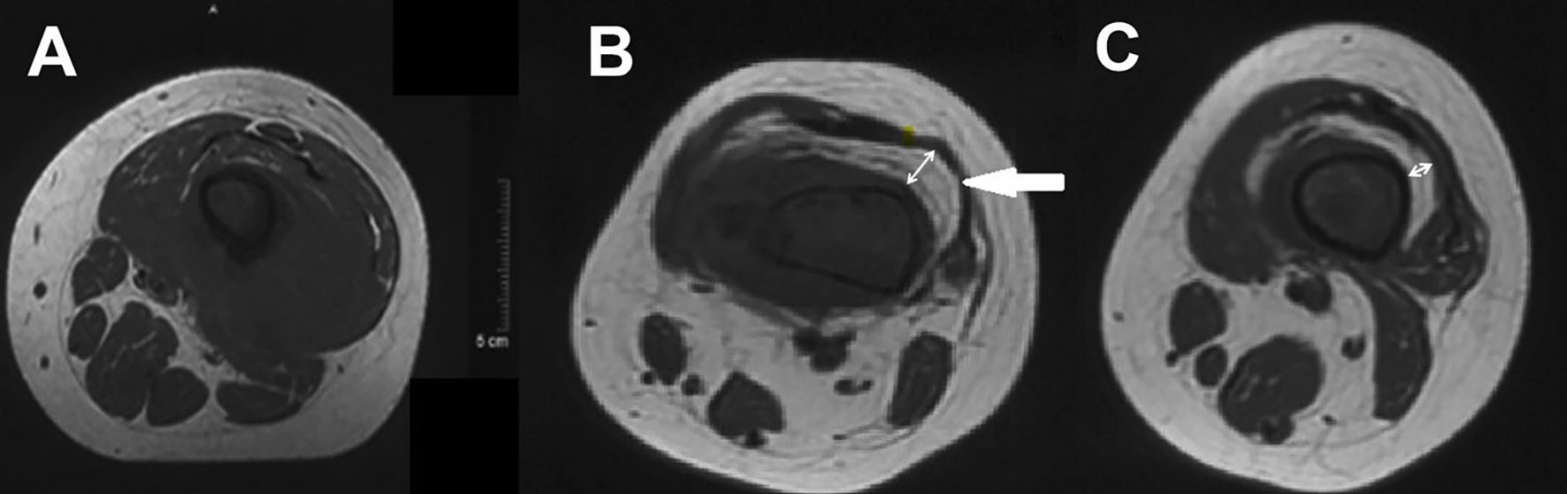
(A) Pre- and (B, C) post-chemotherapy T1-weighted cross-section MR images of a distal femur. The double directional arrow pointing towards the midpoint of the medullary cavity represents the width of fat layer.
